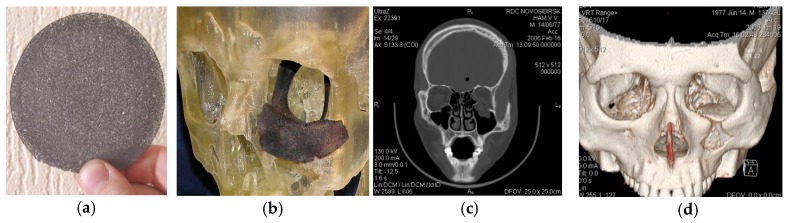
Repair of the orbit: (a) PTN disk plate before modeling, (b) preoperative customization using the 3D printed model; (c) coronal plane CT of the orbit before surgery; and (d) 3D reconstructed postsurgical CT of the repaired orbit [105].
